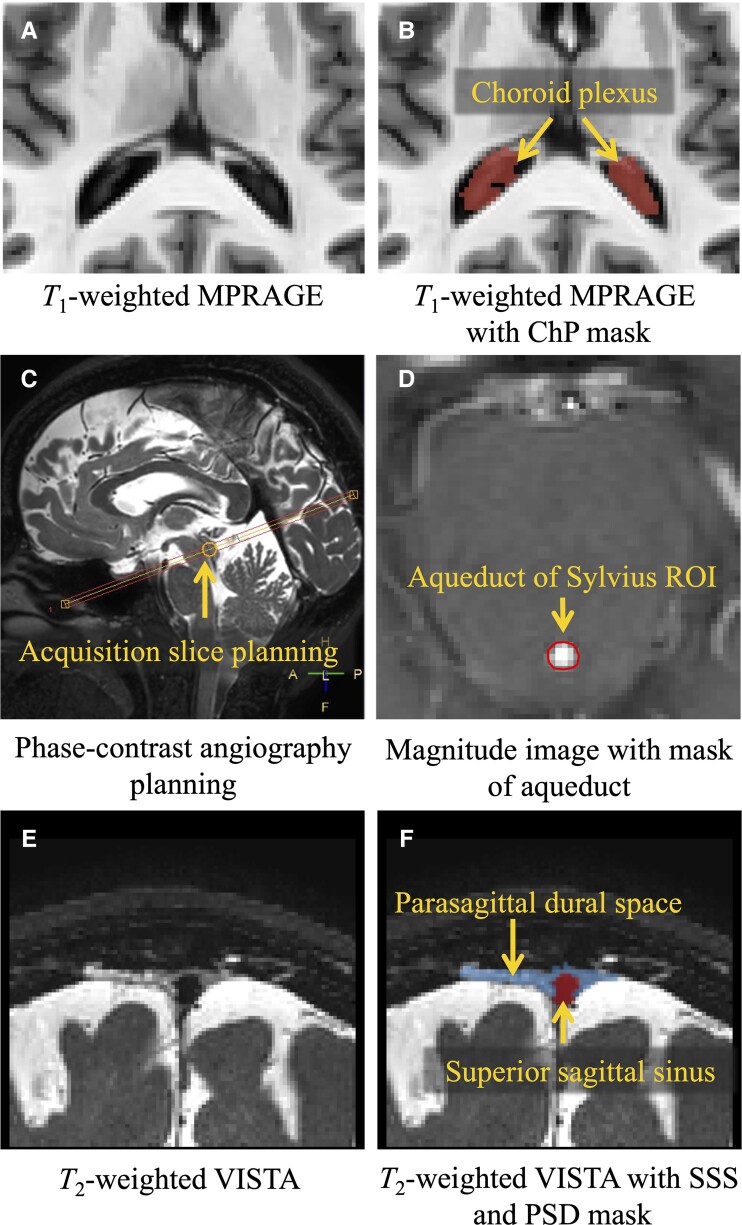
Overview of MR images and processing results. (A and B) Example axial view of a T_1_-weighted MPRAGE image and the resulting ChP (red) mask generated from the F-CNN for automatic segmentation of the ChP. (C and D) Example image of the phase-contrast acquisition planning on a sagittal view of T_2_-weighted VISTA image and an axial view of the magnitude image from acquisition to delineate the cerebral aqueduct (red) for use in the calculation of net CSF flow. (E and F) Coronal view of a T_2_-weighted VISTA image and the output mask (blue, PSD space; red, superior sagittal sinus) from the semi-supervised machine learning method using an F-CNN for automatic segmentation of the parasagittal space.
